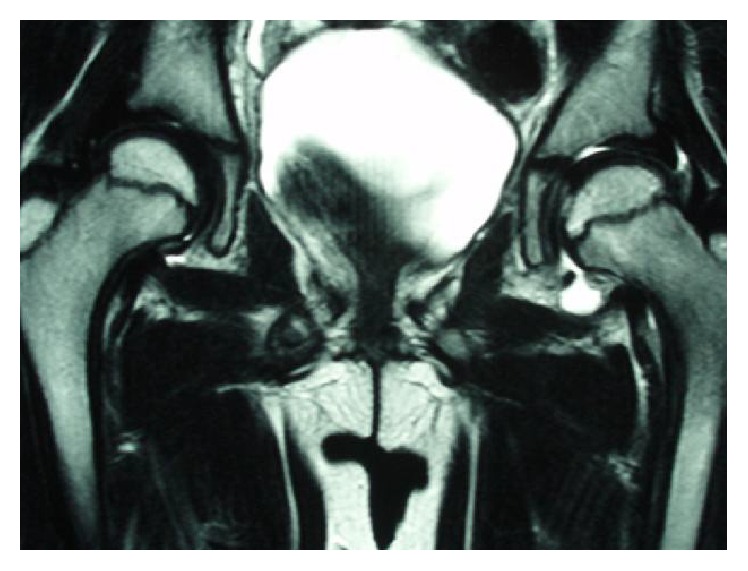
Coronal, T2-weighted magnetic resonance imaging scan at the initial visit. No abnormal findings, except left hip joint effusion, were observed.
